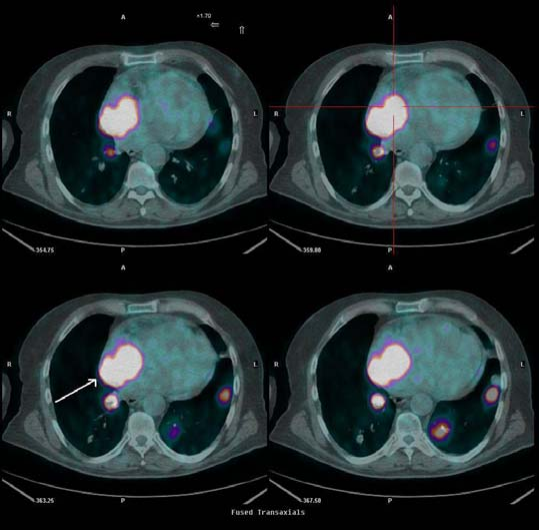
Second [^18^F]FDG-PET/CT scan, performed three months later, showing right atrial uptake (SUV bw max 24) together with pulmonary lesions.
